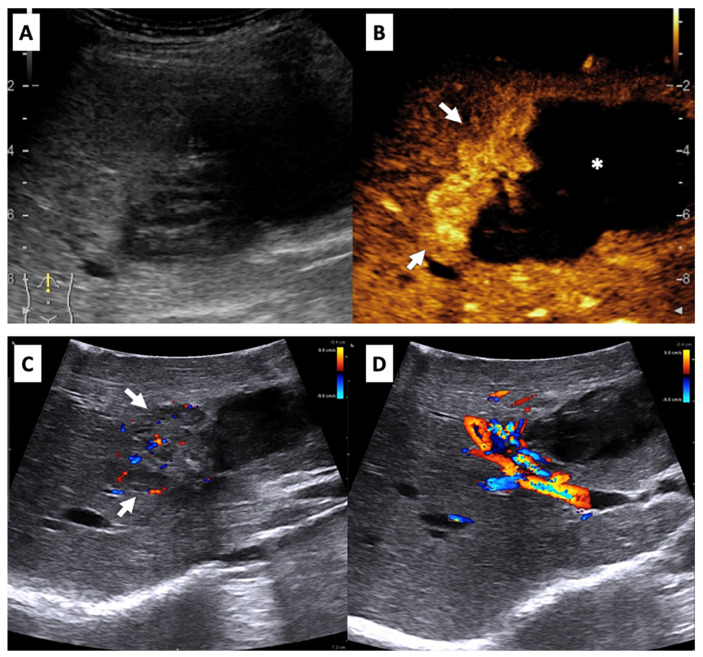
Images of preoperative contrast-enhanced and intraoperative ultrasound. Preoperative conventional (A) and contrast-enhanced ultrasound of the liver, arterial phase (B). A hypervascular ill-defined mass (arrows) in the right liver lobe (B), closely related to avascular heterogenous intraparenchymal liquid collection—hematoma (asterisk). Intraoperative ultrasound (C,D). A heteroechogenic vascularized solid mass in the right liver lobe (C), close to the main right anterior arterial and portal vascular branches (D).
